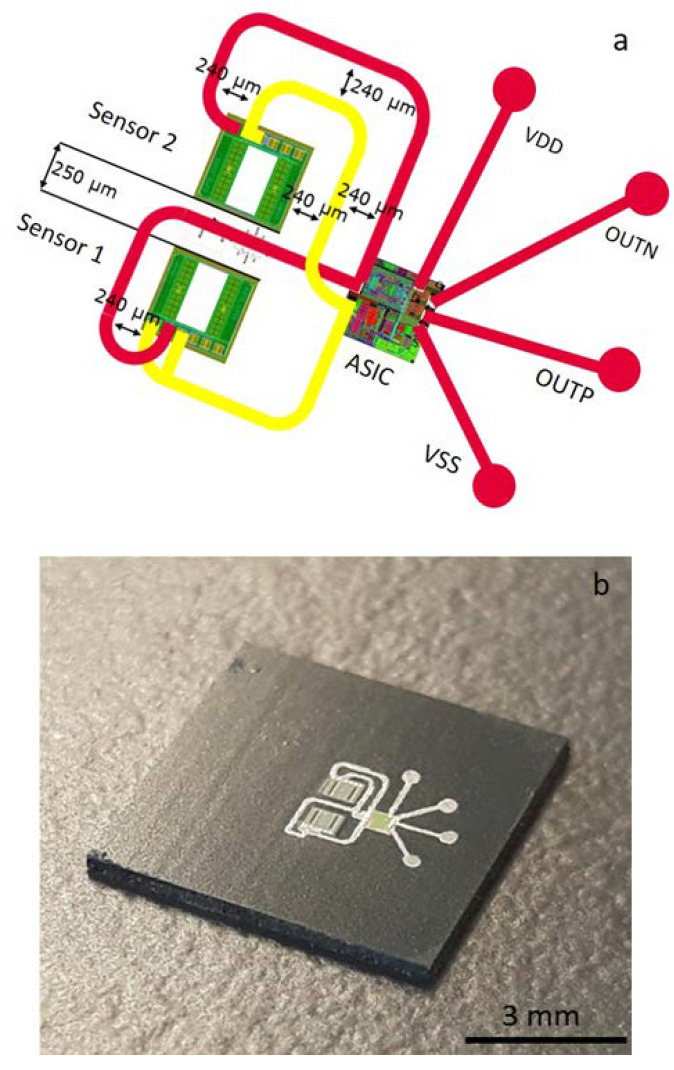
An example for the layout (a) and the final demonstrated FOWLP of two capacitive micromachined ultrasonic transducers (CMUTs) and an application-specific integrated circuit (ASIC) chip with inkjet-printed redistribution layers (RDLs) (Comprised of 2 layers of Ag printed lines (red and yellow) and an intermediate insulating printed layer (not shown)) (b).
